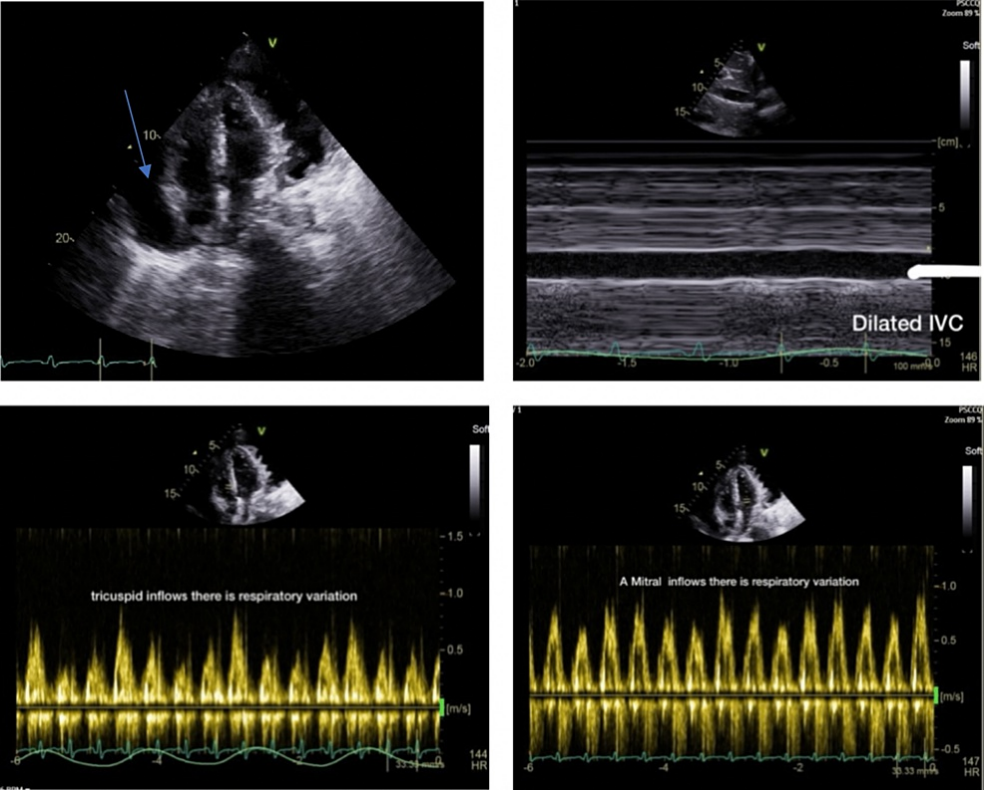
Echocardiography It revealed significant pericardial effusion (blue arrow), and also it showed dilated inferior vena cava (IVC) with respiratory changes in mitral and tricuspid inflows consistent with cardiac tamponade.
